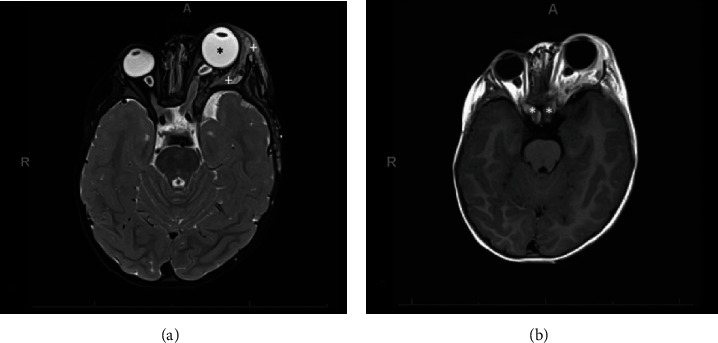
Magnetic resonance imaging. (a) Axial T2-weighted magnetic resonance image shows buphthalmos and exophthalmos of the left eye (asterisks denote the left eye). Also seen are neurofibromas in the left orbit, eyelid, and temple (plus signs denote the tumour). (b) Axial T1-weighted magnetic resonance image shows glioma of both optic nerves with diffuse enlargement (asterisks denote the tumours).
